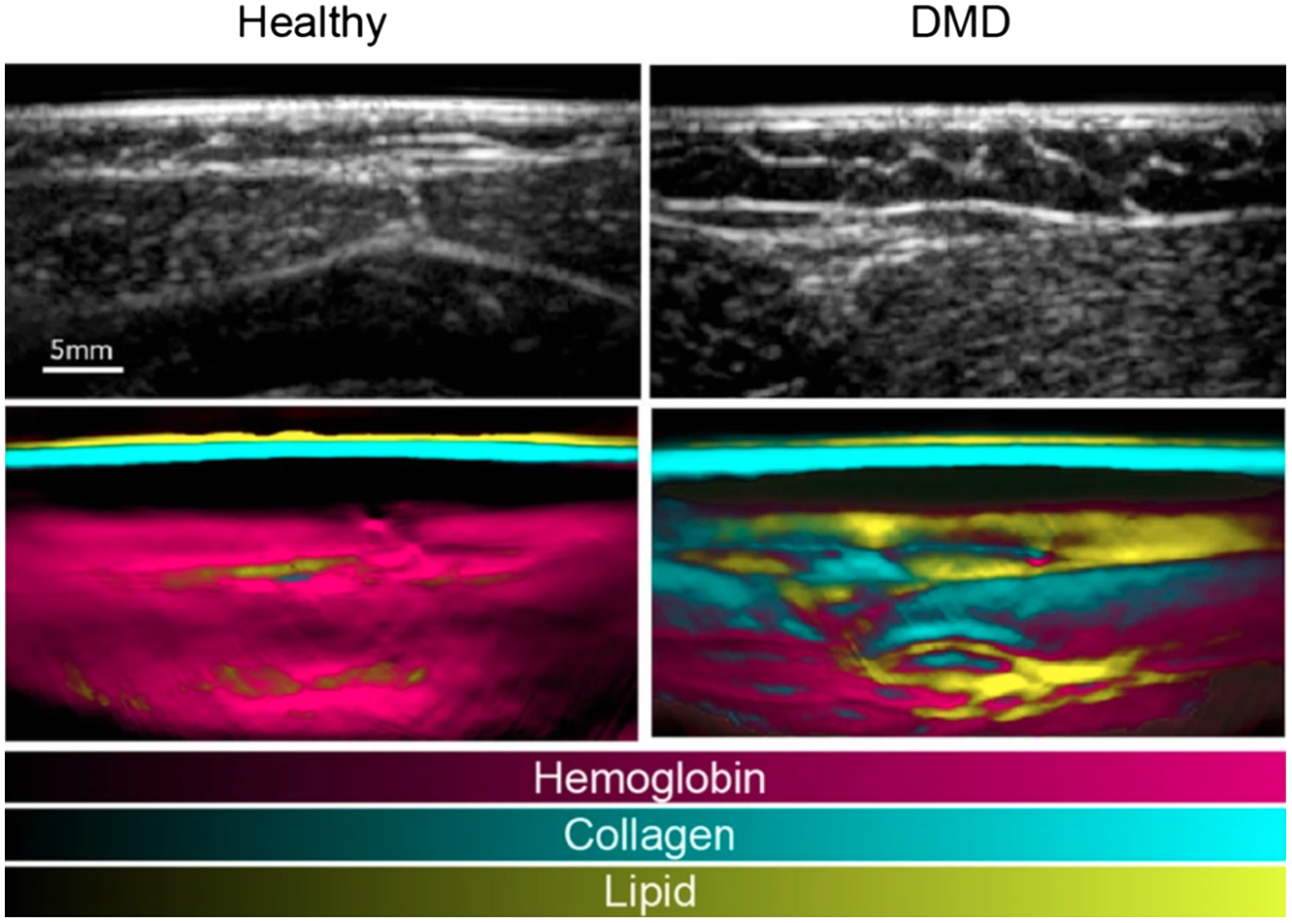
Imaging of muscle collagen as a biomarker for DMD. Top: Ultrasound echography in a healthy volunteer (left) and an individual with DMD (right). Bottom: Multispectral photoacoustic data unmixed for hemoglobin, collagen, and lipid, showing markedly higher collagen content and lipid heterogeneity as markers of DMD. Adapted from Ref. 199 and available under a CC-BY 4.0 license.
